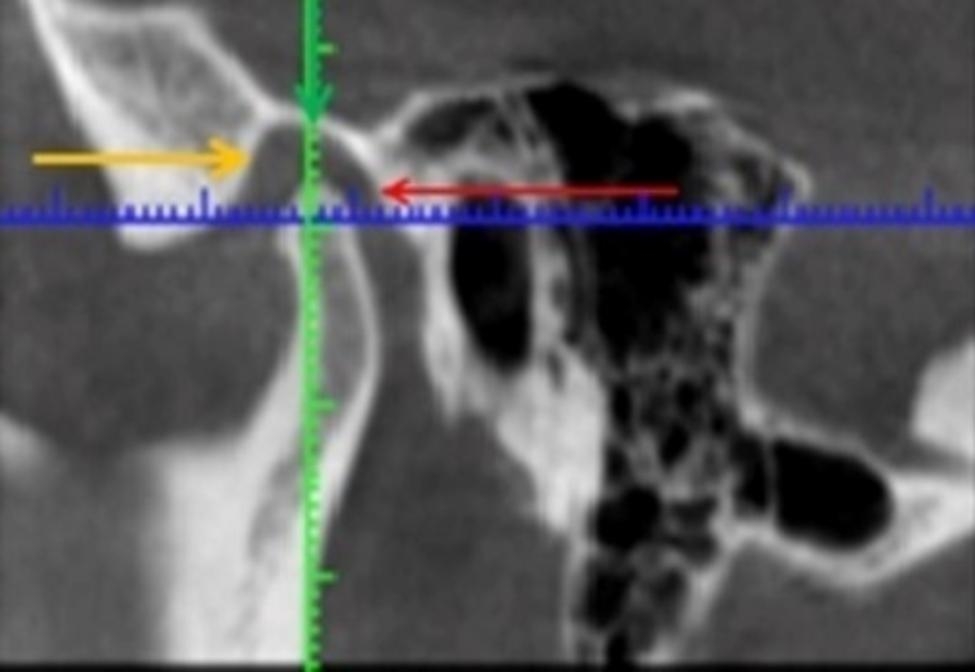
Computer tomography (CT) after 6 months treatment
